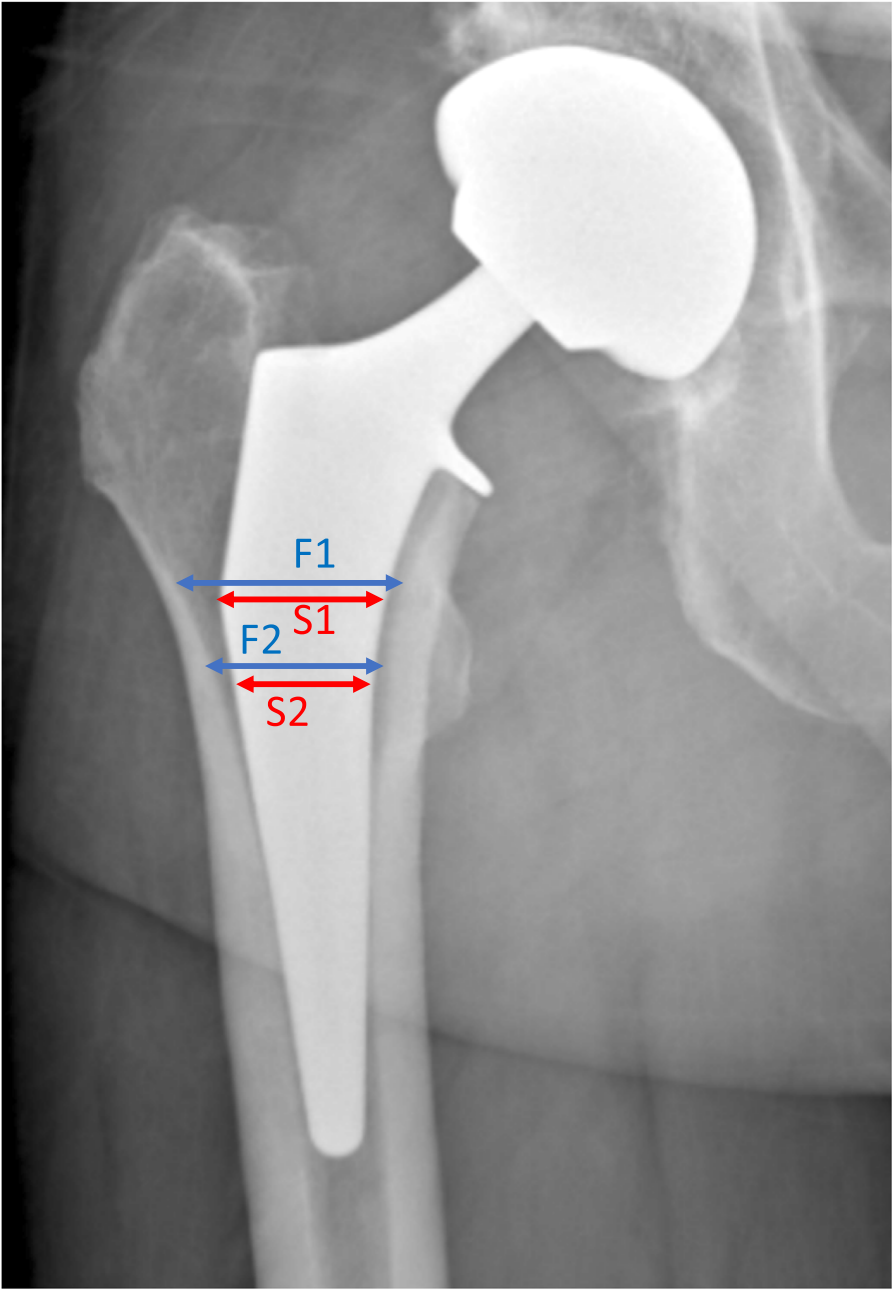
Postoperative radiograph detailing the measurements of canal fill ratio. The blue lines represent the measurement of the width of the inner femoral cortex (F1), and the red lines those of the stem width (S1). The CFR is the ratio between the width of the stem and that of the inner femoral cortex (S1/F1). It is measured at both the upper edge of the lesser trochanter (F1/S1), and at its tip (F2/S2)
